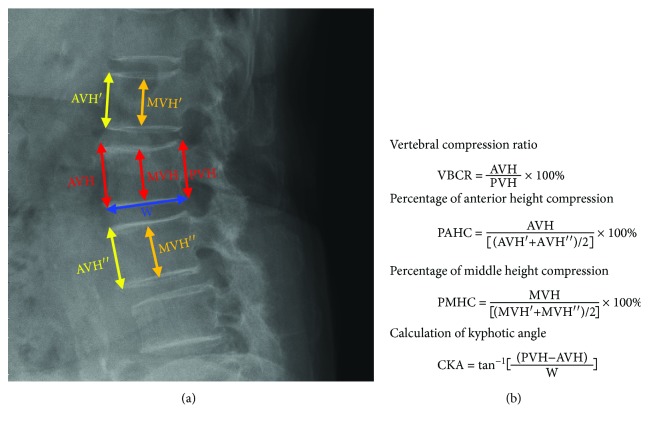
(a) This study mainly measures the characteristic lengths on plain radiographs (AVH: anterior vertebral height; MVH: middle vertebral height; PVH: posterior vertebral height; W: vertebral body width). (b) Parameters examined in this study (VBCR, PAHC, PMHC, and CKA).
